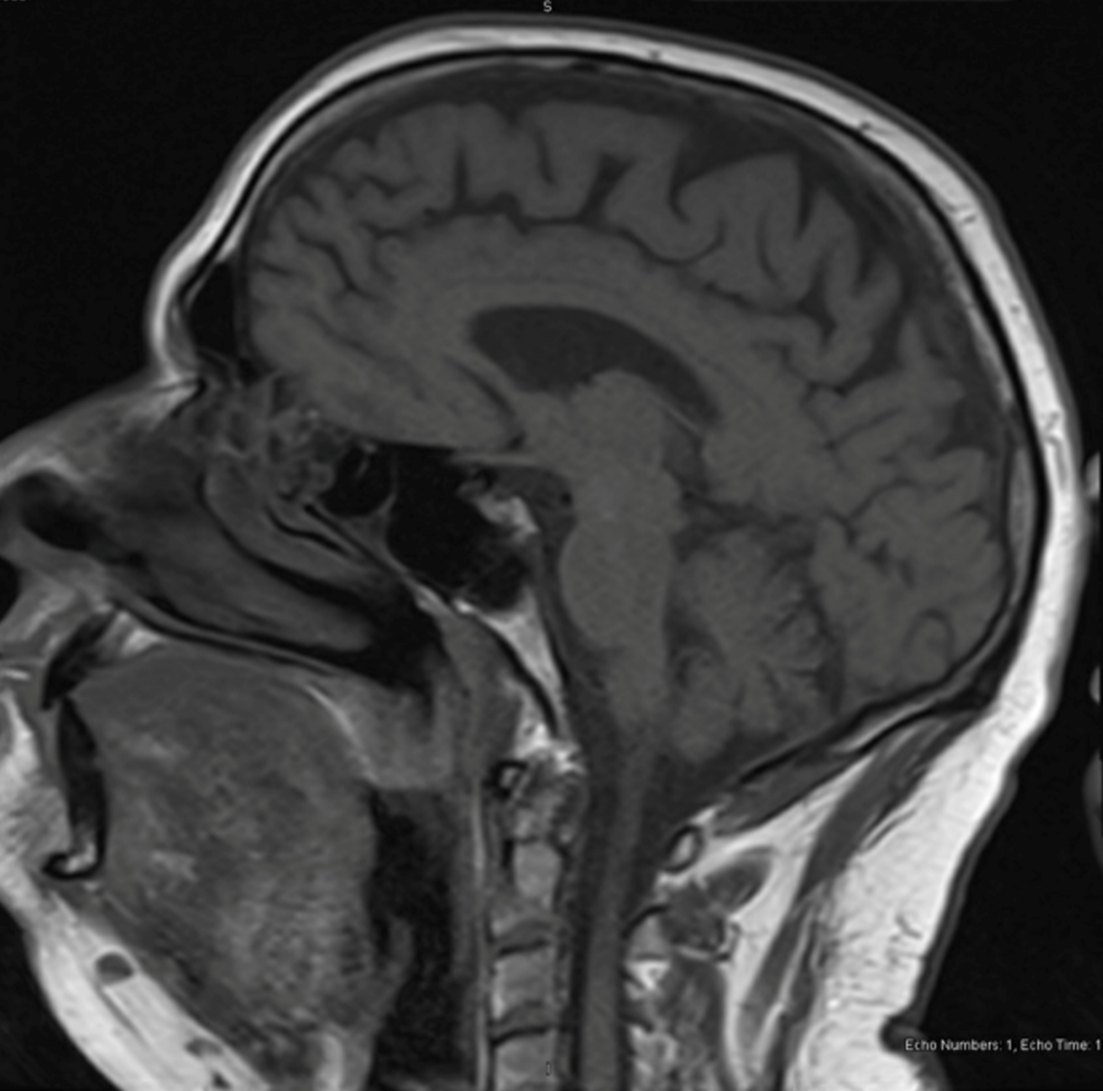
Sagittal MRI after resolution of patient's symptoms with no acute findings
